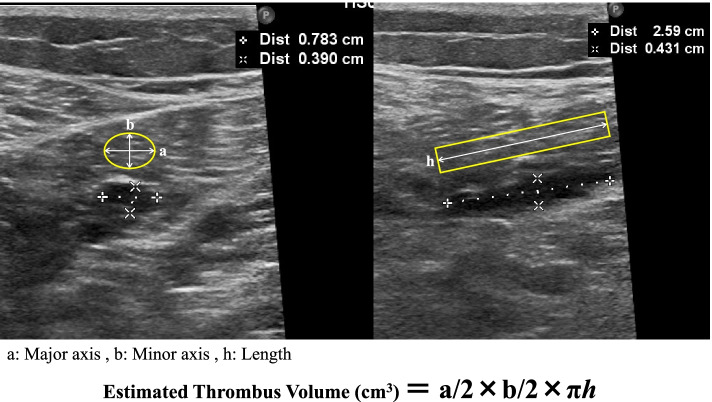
Method for estimation of thrombus volume. Thrombus volume was calculated by approximation of the value determined by ultrasonography to an elliptic cylinder [18]. a: Major Axis, b: Minor axis, h: Length. Estimated Thrombus Volume (cm3) = a/2 × b/2 × πh
